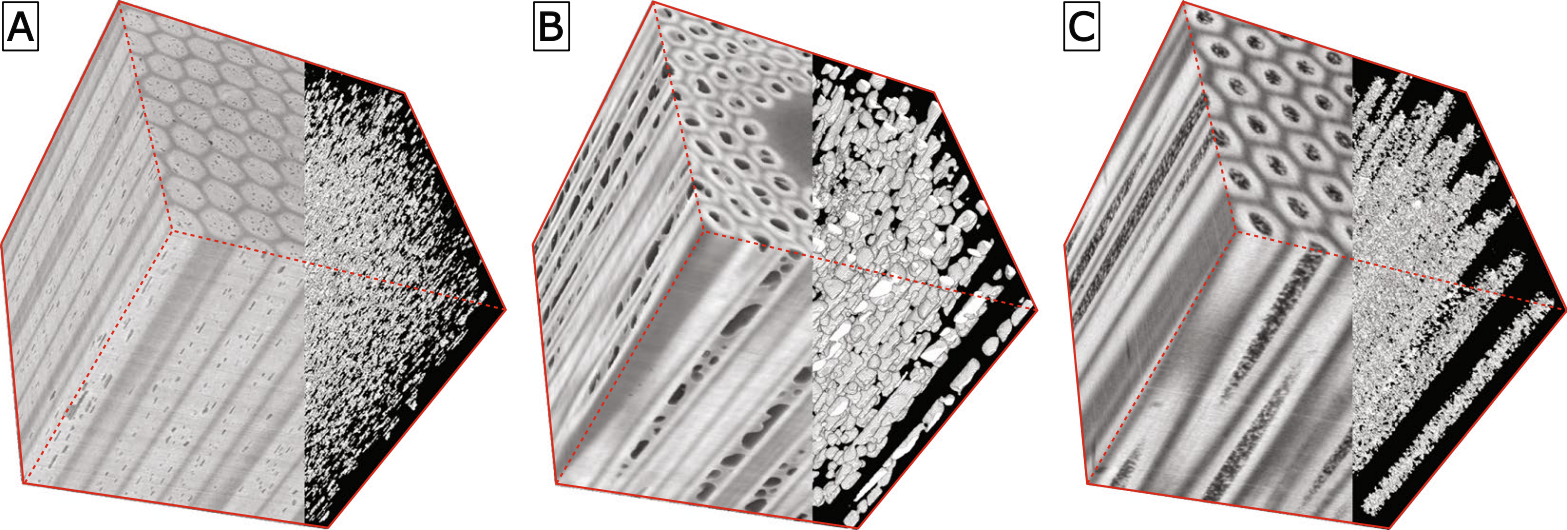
Exemplary 3D assembled X-ray tomography images in a cube of Bronze (A) RRP (B) and PIT Nb_3_Sn wires (C).
